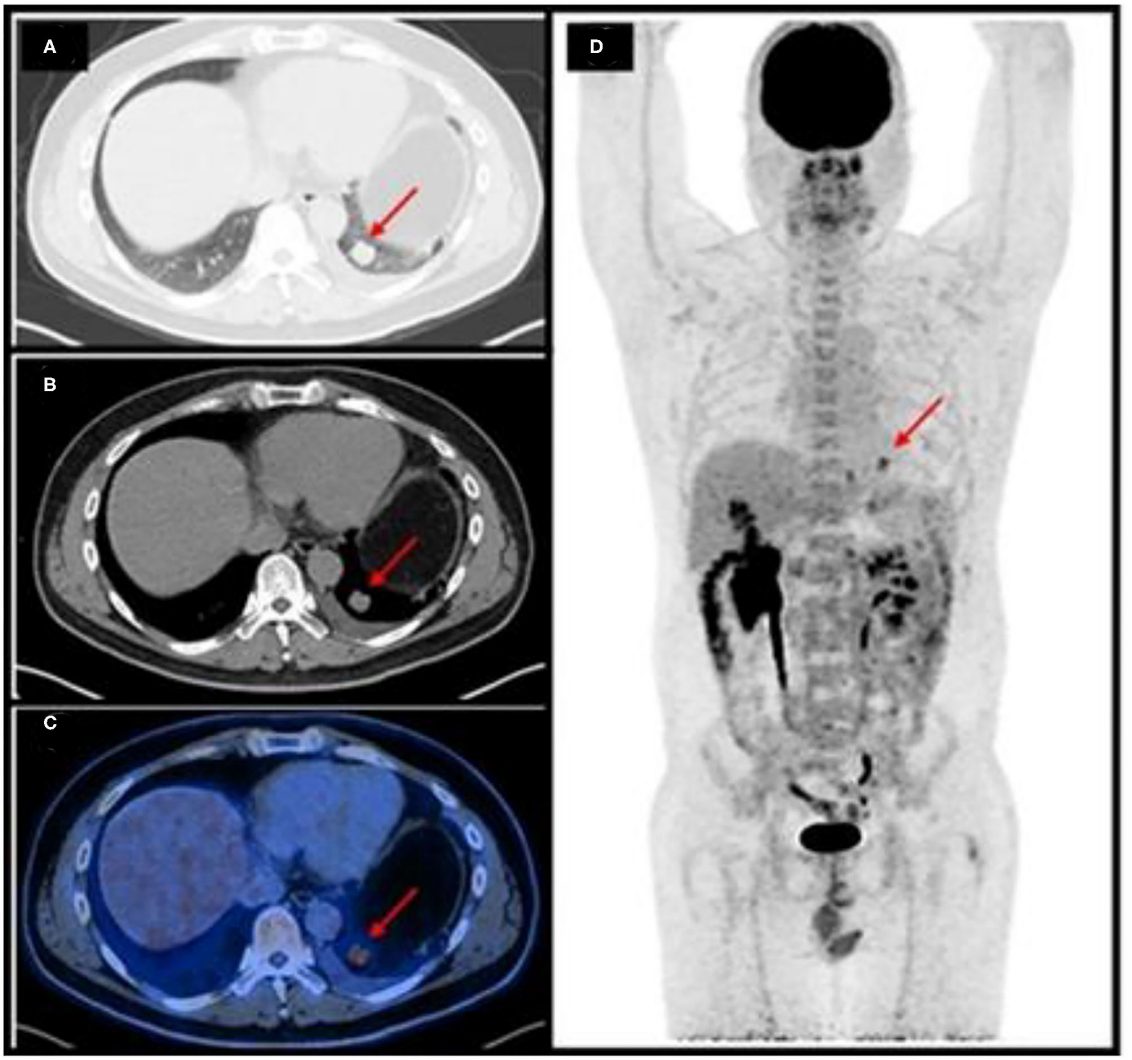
Initial position emission tomography/computed tomography (PET/CT) imaging showed a mildly hypermetabolic lesion in the inferior lobe of the left lung (arrows). (A,B) axial CT; (C) axial fused PET/CT; (D) maximum intensity projection.
